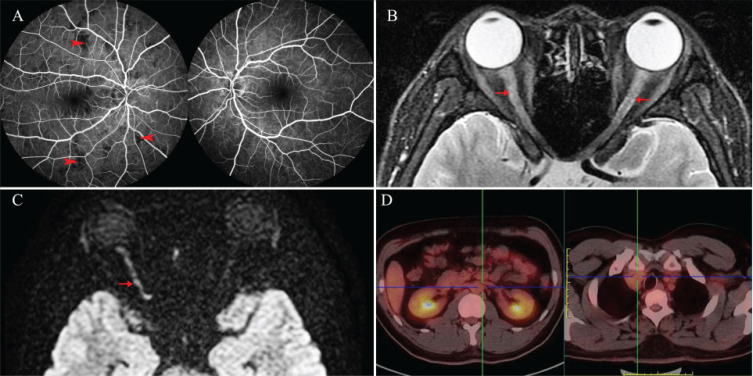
A 32-year-old man with Takayasu’s arteritis. A: FA: Bilateral disc edema without fluorescein leakage. Several choroidal ischemic lesions are present in the right eye (red arrowhead). B: Axial T2-weighted MRI: Discrete T2-weighted hyperintense in both optic nerves and sheaths (red arrow). C: DWI: Mild restricted diffusion in the right optic nerve (red arrow). D:
